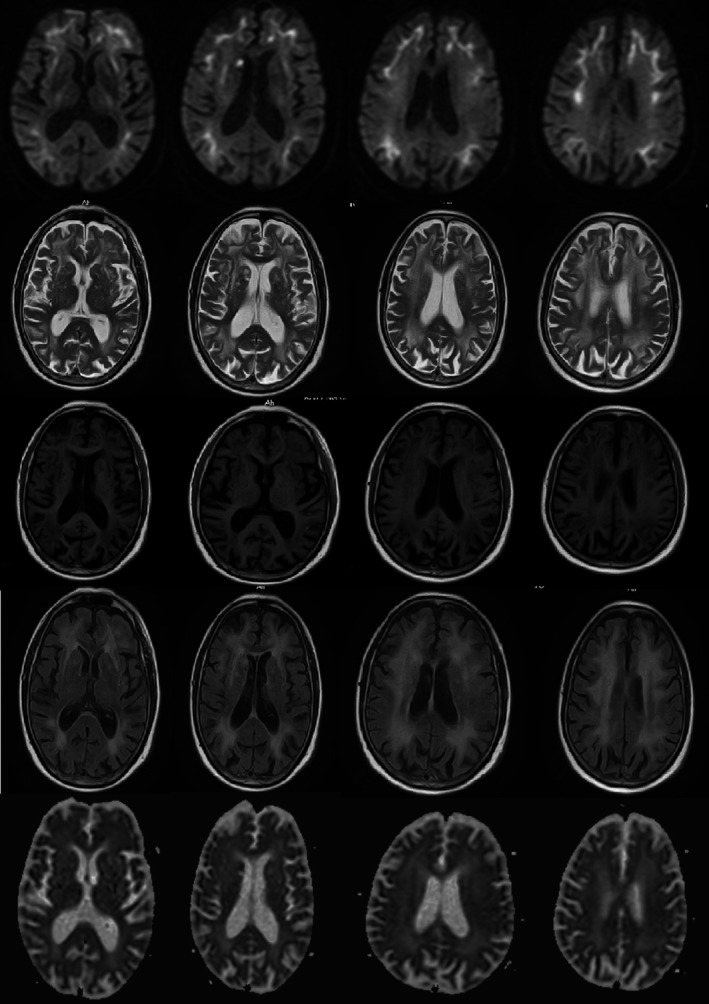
Diffusion‐weighted magnetic resonance imaging reveals symmetrically distributed strip‐shaped isointense signal in bilateral fronto‐occipital‐parietal cortical‐medullary junction, with nodular hyperintense signal in the right paraventricular white matter. The corresponding lesions in the cortical‐medullary junction showed isointense or slightly hyperintense signals on ADC sequence, and the corresponding lesions in the paraventricular region exhibited hypointense signal on ADC sequence. Multiple patchy hyperintense signals in bilateral paraventricular region, corona radiata, and semioval center were displayed on T2. The corresponding lesions showed hypointense signals on T1 sequence and hyperintense signals on Flair sequence
